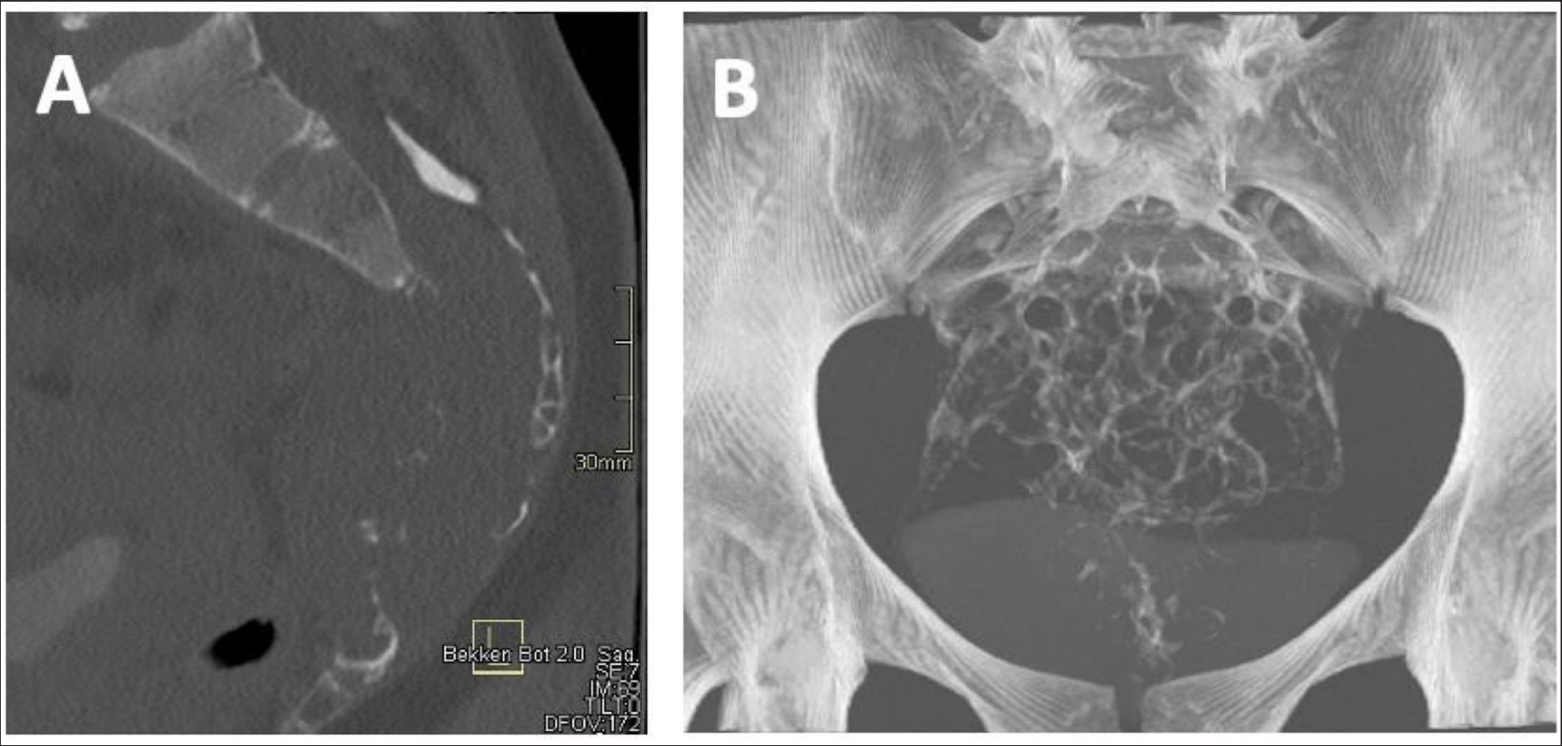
(A) Midsagittal bone-window CT; (B) Coronal MIP CT.
